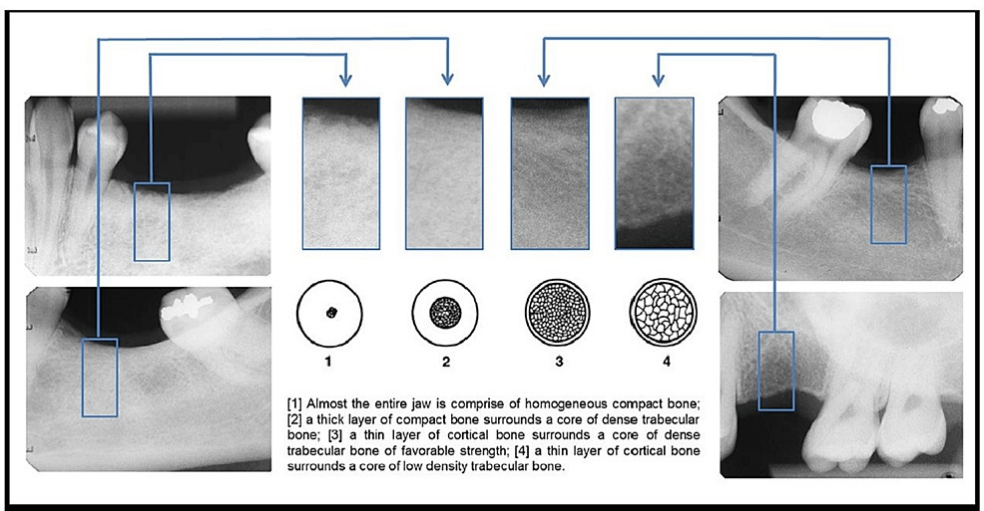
Jawbone site radiographic images according to Lekholm and Zarb’s bone classification
